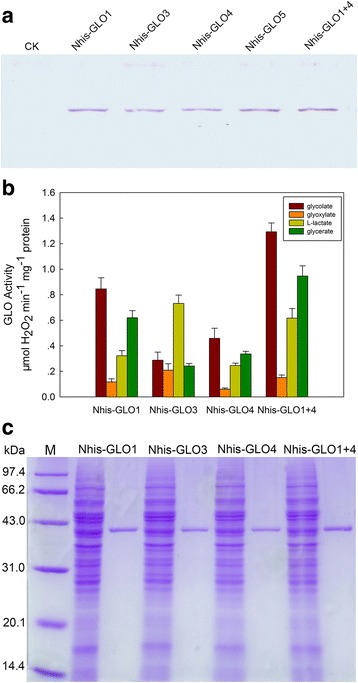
Expression of GLO isoforms in yeast. a Western blotting analysis of each GLO isoform expressed in yeast. CK represents the crude enzyme extracted from yeast cells transformed with pYES3/CT vector. Nhis-GLO1, Nhis-GLO3, Nhis-GLO4 and Nhis-GLO5 represent the crude enzyme extracted from yeast cells transformed with pYES3-Nhis-GLO1, pYES3-Nhis-GLO3, pYES3-Nhis-GLO4, pYES3-Nhis-GLO5 respectively. Nhis-GLO1 + 4 represent the crude enzyme extracted from yeast cells co-transformed with pYES3-Nhis-GLO1 and pYES2-Nhis-GLO4. b Substrate specificity of each GLO isoform. Values are means ± SD (*n* = 3). c The purity and subunit molecular weights of the GLO isoforms purified from yeast. GLO isoforms were purified from yeast cells by immobilized metal affinity chromatography, and the molecular weights of the subunits were determined by uniform SDS-PAGE (12.5%). The results are representative of three independent experiments
